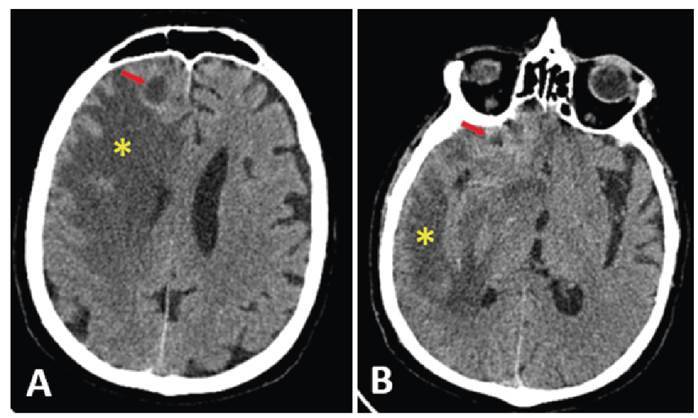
1: A 68-year-old male patient with intracranial aspergillosis. A-B: The axial non-contrast brain CT shows centrally hypodense lesions with peripheral hyperdense areas, compatible with an abscess (red arrows), in the anterior part of the right frontal lobe. Additionally, there is extensive vasogenic edema in the surrounding brain
